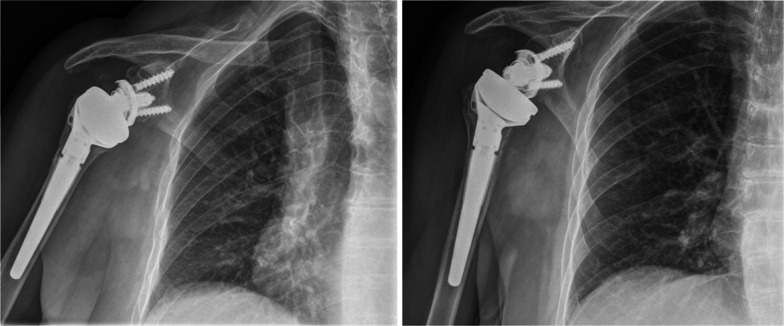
Postoperative X-rays after reverse shoulder arthroplasty
